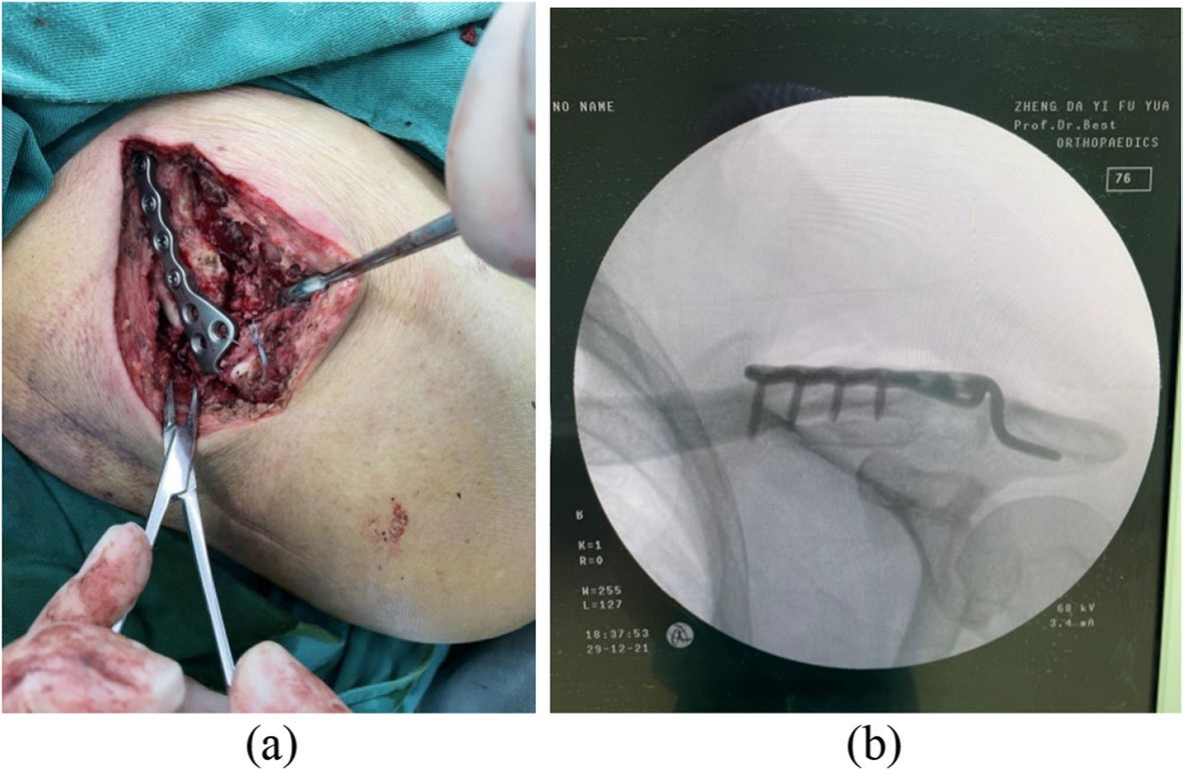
a: Dislocation of the ACJ with skin incision at all levels, exposure of the ACJ, and implantation of the clavicle hook plate, which can be seen as a surgical incision about 10 cm long; b: The C-arm X-ray device showed that the reduction of ACJ was good
